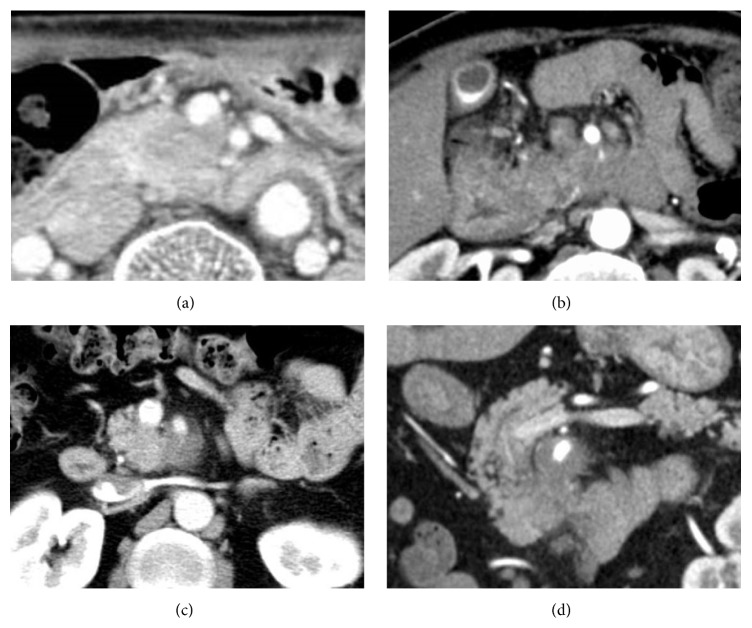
Representative computed tomography images of borderline resectable pancreatic cancer and locally advanced unresectable pancreatic cancer. (a), (b) Tumor in the pancreatic head slightly abutting the portal vein and invading less than half of the circumference of the superior mesenteric artery (borderline resectable pancreatic cancer). (c), (d) Tumor in the pancreatic head invading less than half the circumference of the superior mesenteric artery (locally advanced unresectable pancreatic cancer).
